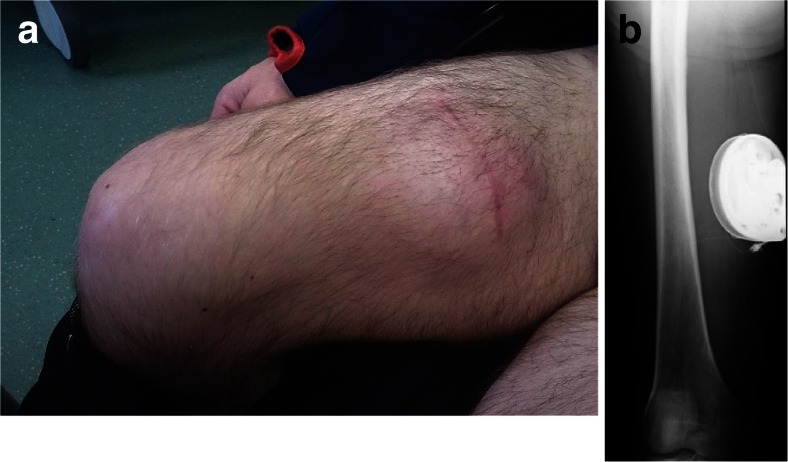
a Subcutaneous location of intrathecal baclofen pump device in the right medial thigh 5 months after surgery. b Radiograph showing the subcutaneous placement of the intrathecal baclofen pump device with associated catheter located in the right medial thigh. The catheter is seen passing superiorly from the device towards the right neck of femur
